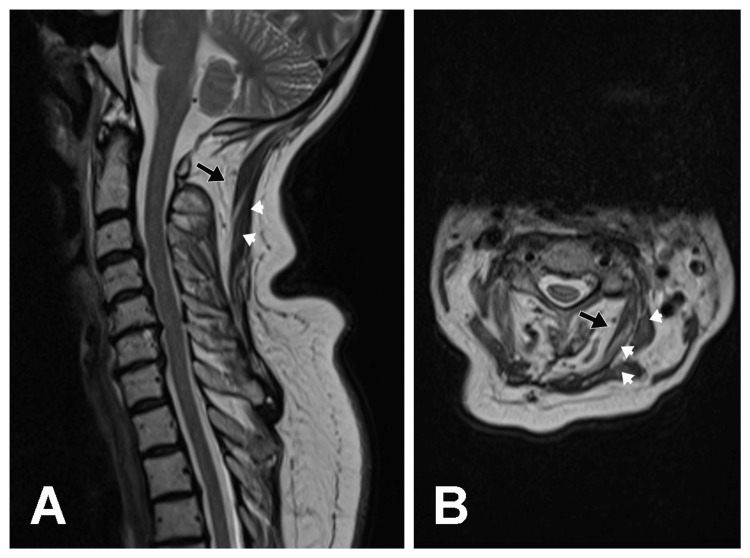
Sagittal and Axial T2-Weighted MRI Images of the Cervical Spine (A) Sagittal and (B) axial T2-weighted MRI images of the cervical spine demonstrating extensive fatty degeneration of paraspinal muscles (black arrows) and atrophic paracervical muscles containing white areas of fatty degeneration (white arrowheads).  Also note the radiation-induced fatty marrow replacement changes in the cervical spine.
